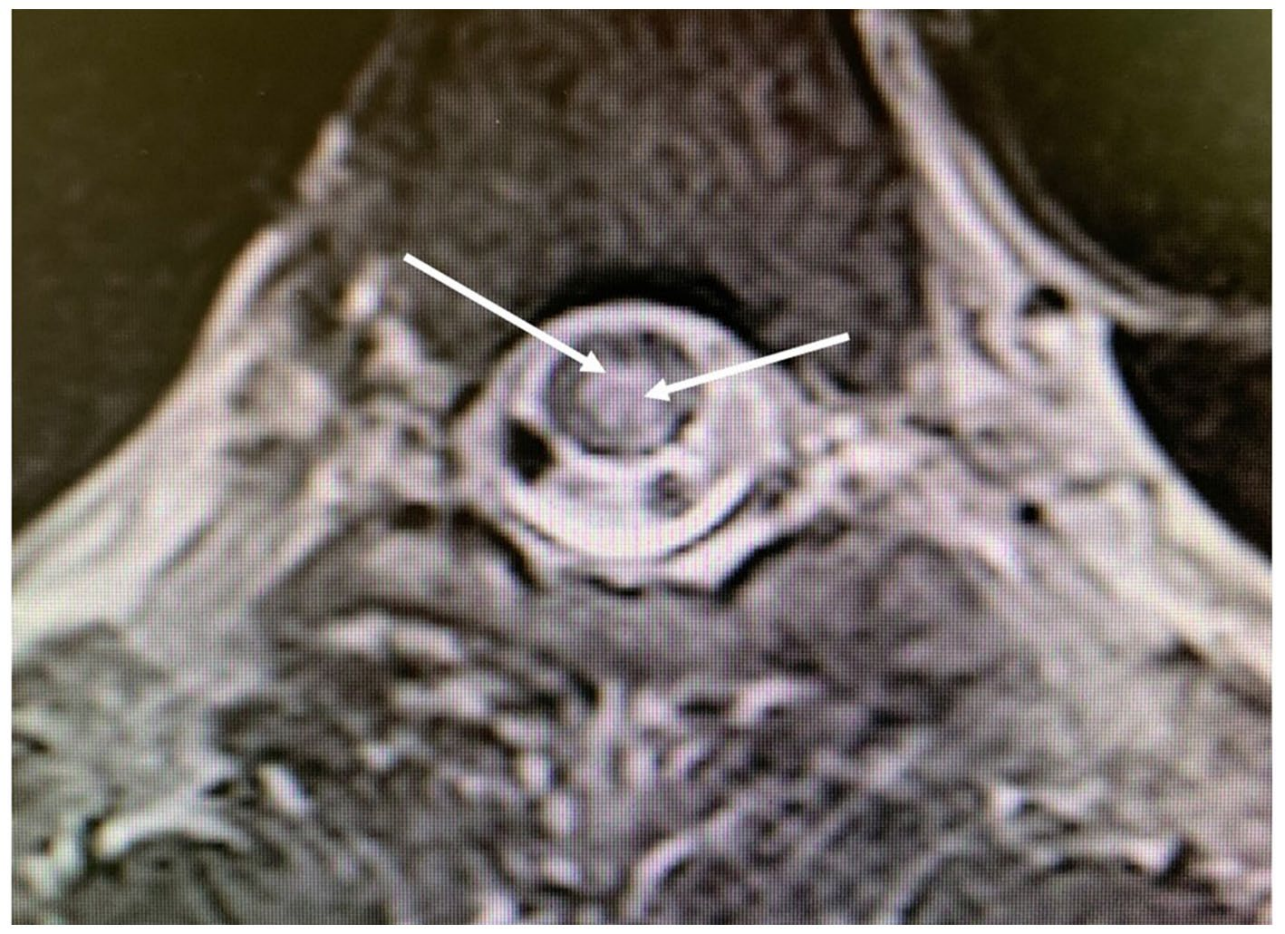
Magnetic resonance imaging of the thoracolumbar spinal cord; axial T2-weighted images obtained 5 days after the second diving incident display abnormalities in spinal cord signal intensity (indicated by arrows), with involvement of the posterior medulla and gray matter.
